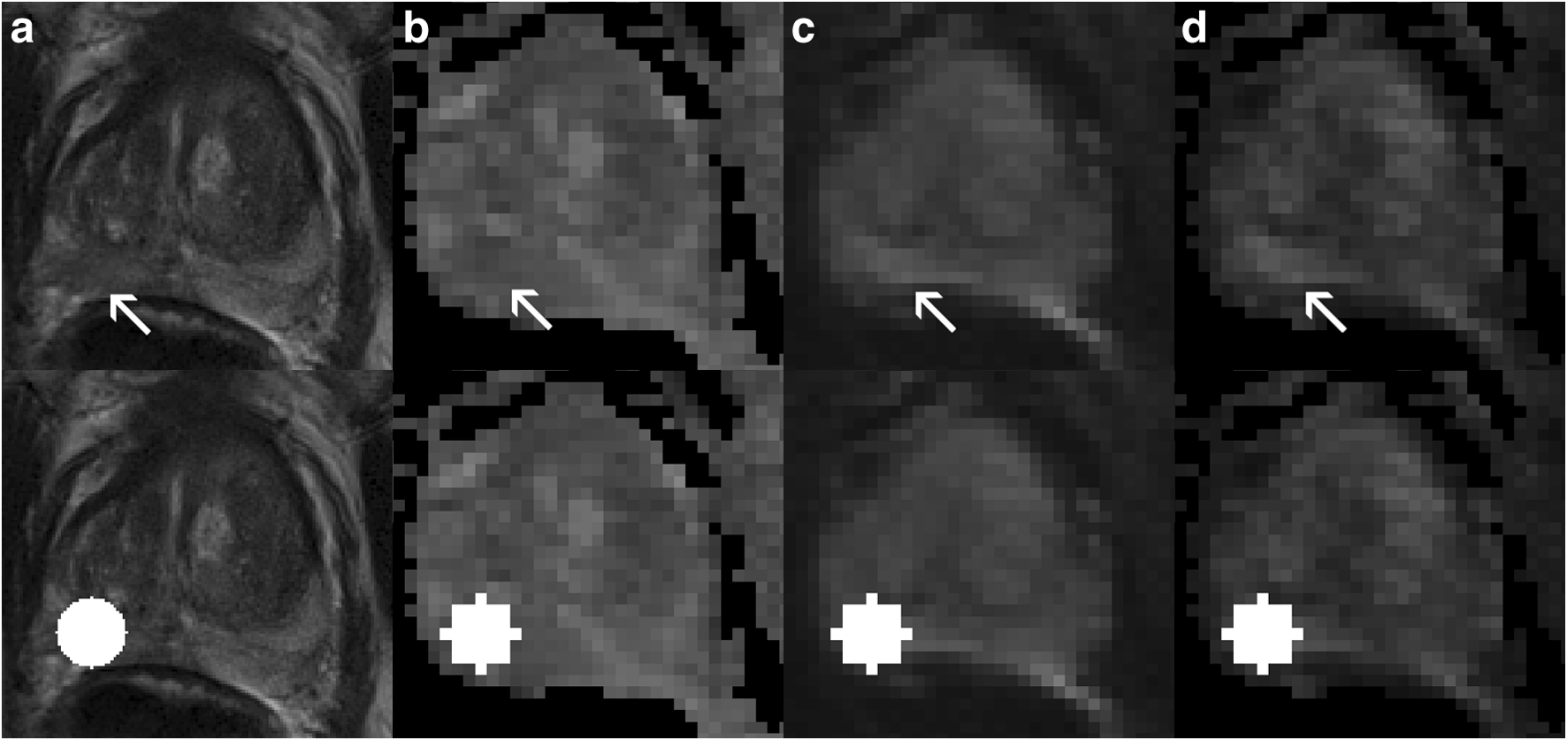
True-positive example for model 3 (T2-w + DWI) which predicted a clinically significant (CS) prostate cancer (PCa) lesion. This patient had a peripheral zone (PZ) lesion (classifiable as PI-RADS 4) which was pinpointed (the visually most aggressive part) originally by an expert (arrow, first row), which proved to be CS PCa (Gleason score > 6). a T2-w (axial), b ADC, c DWI *b*-value 800 s/mm^2^, d DWI calculated *b*-value 1400 s/mm^2^. Second row, segmentations using the auto-fixed volume of interest (VOI, marked in white) were placed around the visually most aggressive lesion pinpoint
